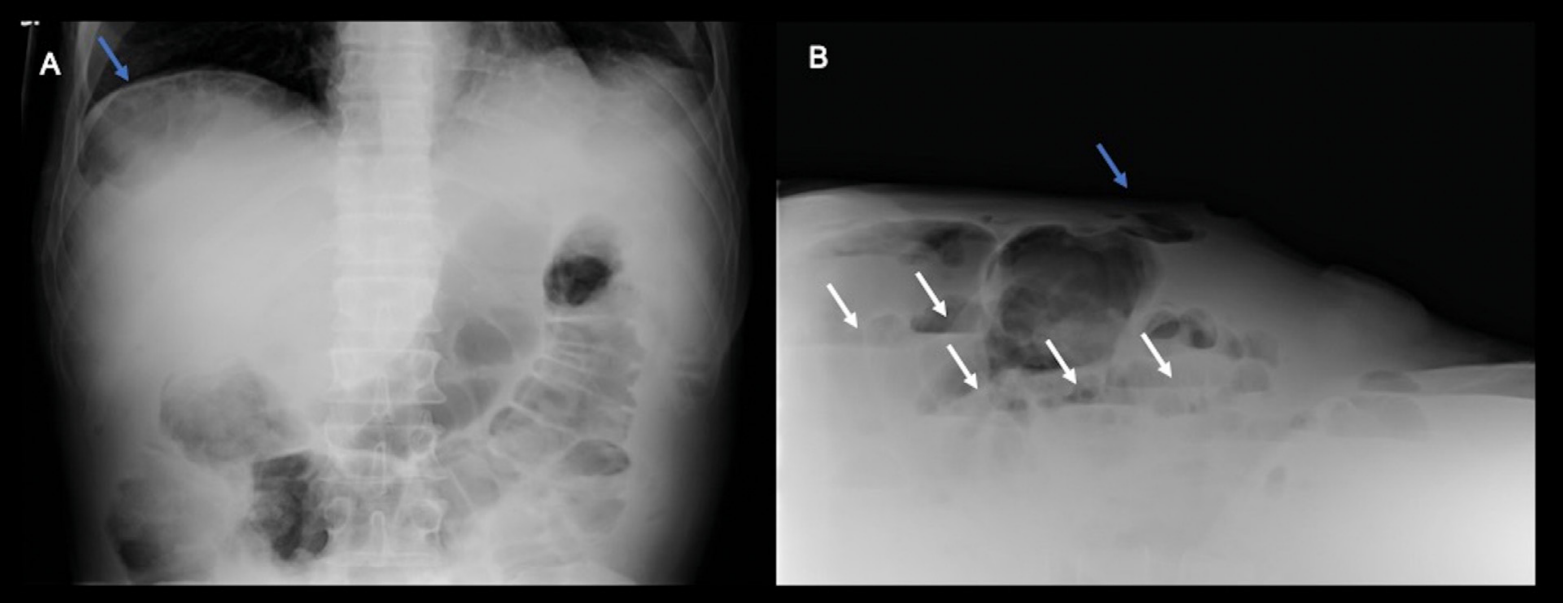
Supine frontal and cross-table lateral view radiographs of the abdomen. Free gas (blue arrows) is seen beneath the right hemidiaphragm on the frontal view and in the non-dependent anterior abdominal peritoneal space on the cross-table lateral view. Also note gas distension of the stomach, duodenum and bowel loops, most of them presenting air-fluid levels (white arrows).
